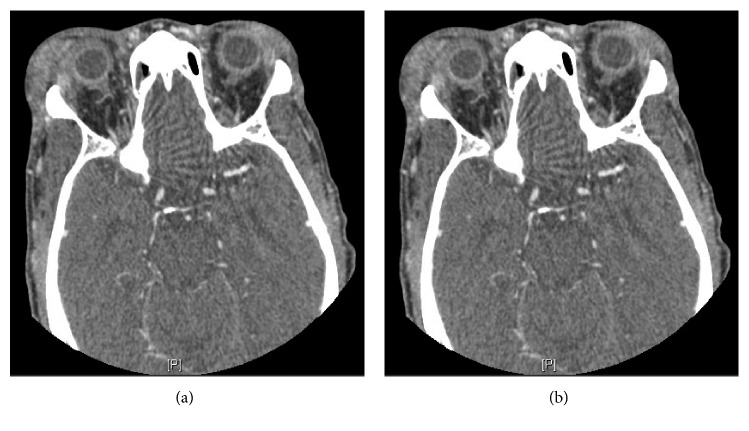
CT orbits with contrast demonstrating enlargement and inflammation within the lacrimal glands and the surrounding tissues: (b) greater than (a).
